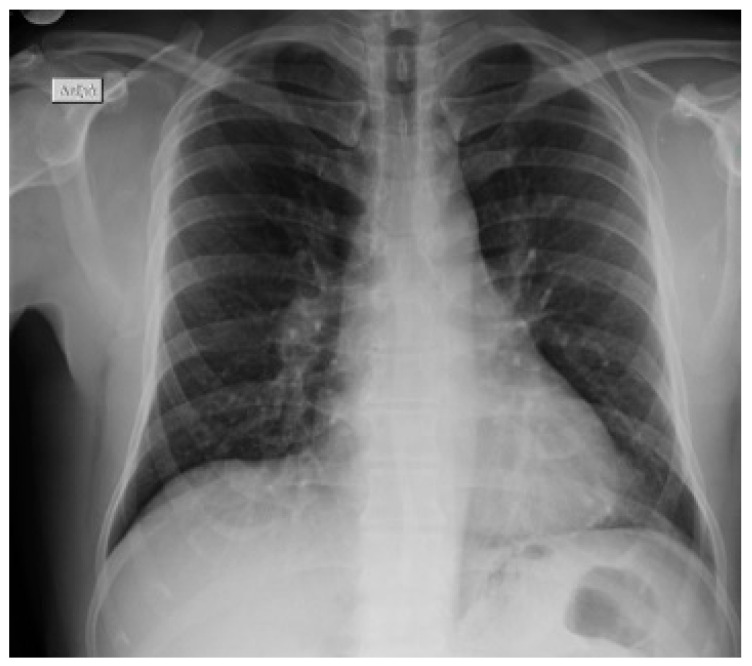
Patient’ s X-ray after he was admitted to the emergency department.
